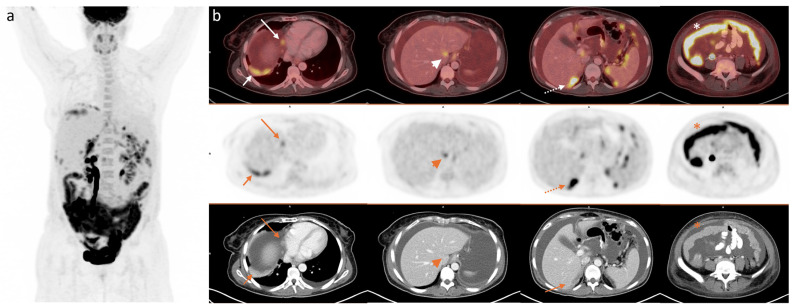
2-[^18^F]FDG PET/CT scan of a 68-year-old woman with AOC prior to first-line treatment showing (a) maximum intensity projection (MIP) and (b) axial images of fused PET/CT (upper), PET (middle), and CT (lower row) with peri-hepatic carcinomatosis (arrows), liver hilum disease (head arrow), Morison pouch implant (dotted arrow), and omental cake (*).
